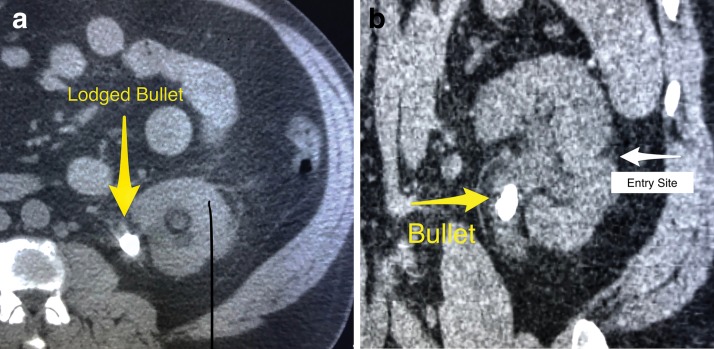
CT scan image indicates a bullet fragment in the right UPJ (a, transverse view; b, sagittal view). UPJ = ureteropelvic junction.
